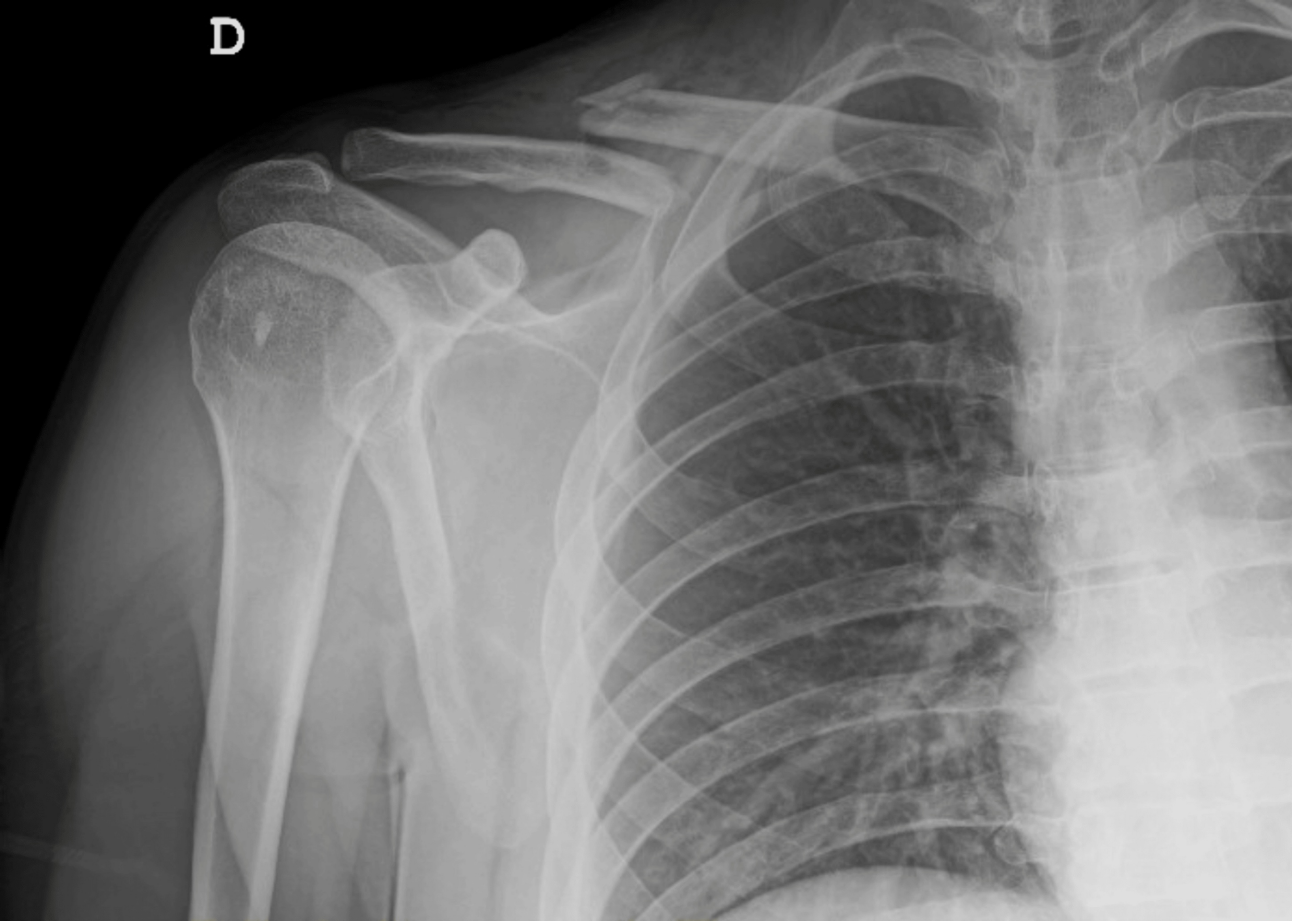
Preoperative X-ray image of right clavicle fracture resulting from trauma
